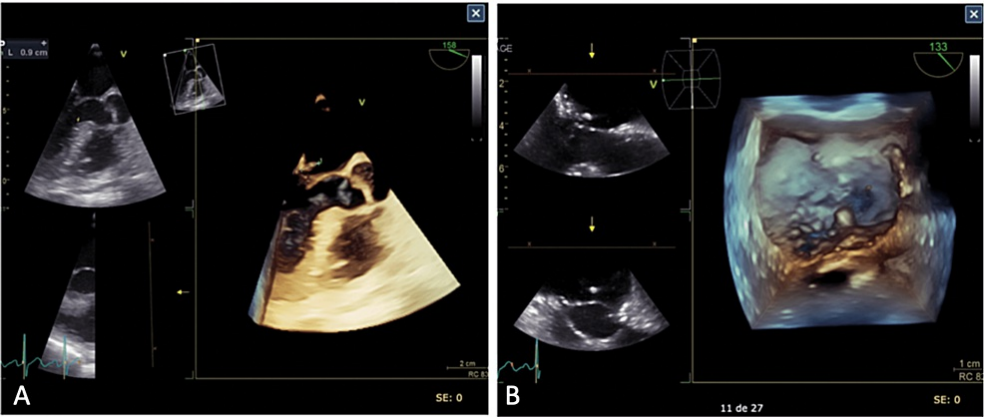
Transesophageal echocardiogram revealing Parvimonas micra vegetation 1A: 3D transesophageal ultrasound showing vegetation on the posterior mitral leaflet with marked mobility towards the auricular surface. 1B: Same sonography with a different projection revealing vegetation on P2
